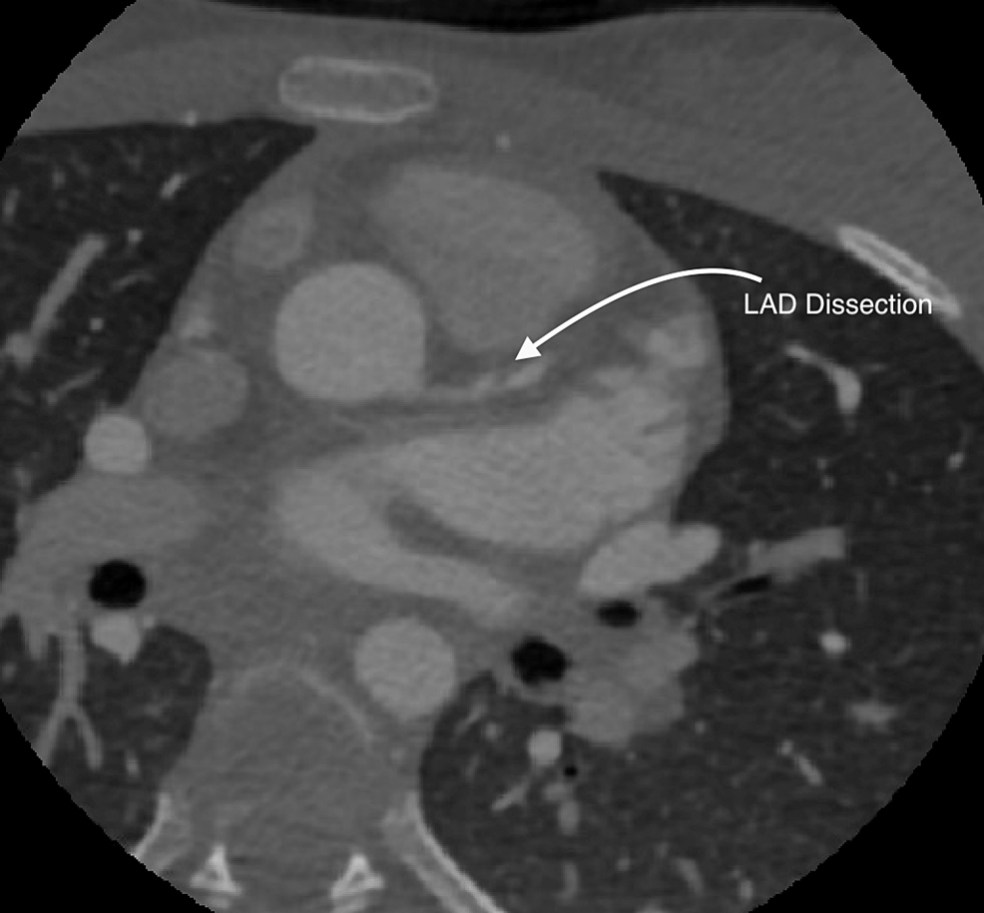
Cardiac CT angiogram showing focal narrowing of left anterior descending artery concerning for possible dissection flap CT: computed tomography
